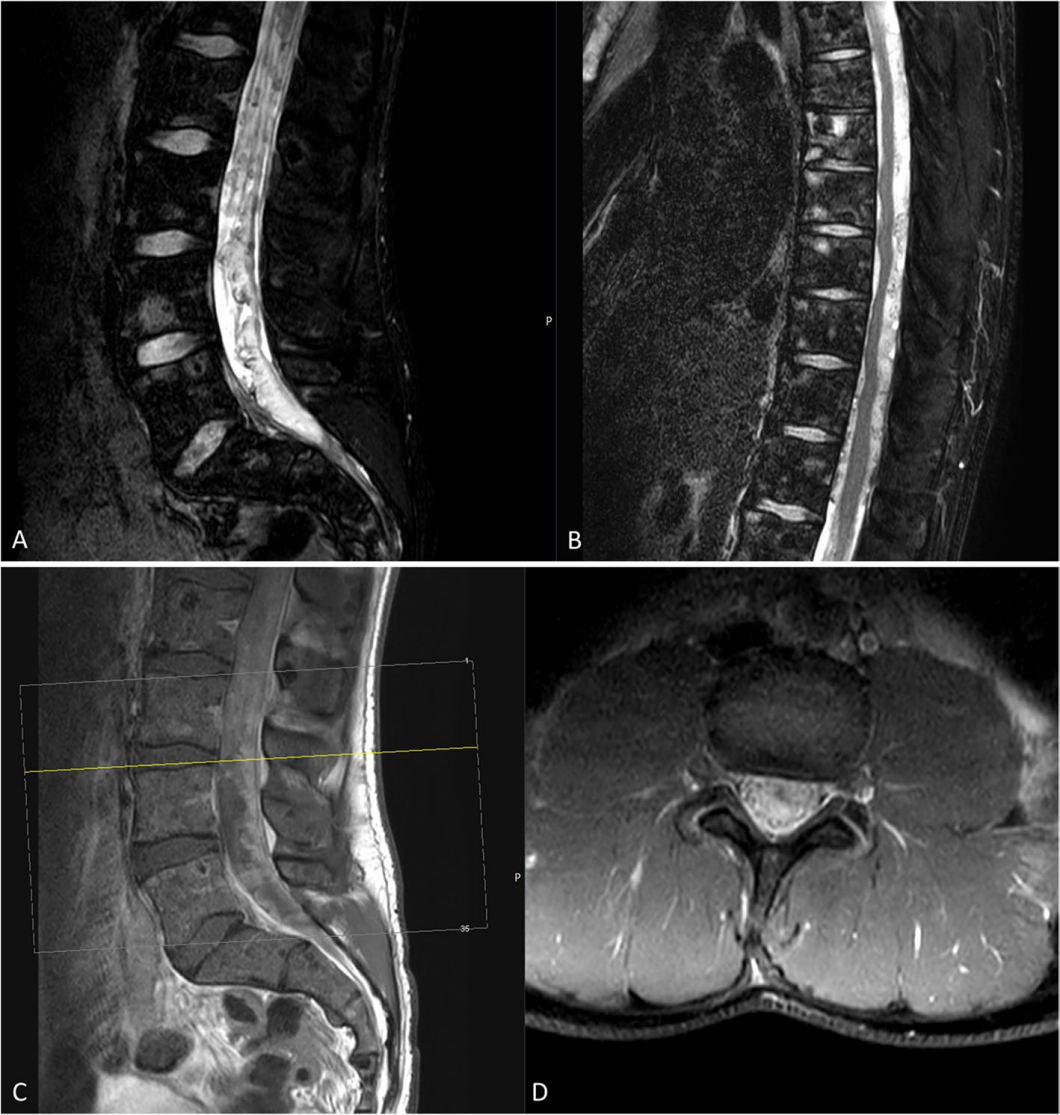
MRI of the thoracic and the lumbar spine. A: sagittal T2-weighted MR-image of the lumbar spine demonstrates diffuse metastatic lesions in the conus medullaris and along the cauda equina. B: sagittal T2-weighted MRI shows diffuse intradural, extramedullar bulky disease along the thoracic spinal cord. Both images of the thoracic and the lumbar spine present intraosseous tumor lesions. C, D Contrast-enhanced T1-weighted sagittal (C) and axial (D) MRI demonstrates diffuse infiltrating bulky tumor-lesions intradural along the spinal cord, the conus medullaris, and the cauda equina
